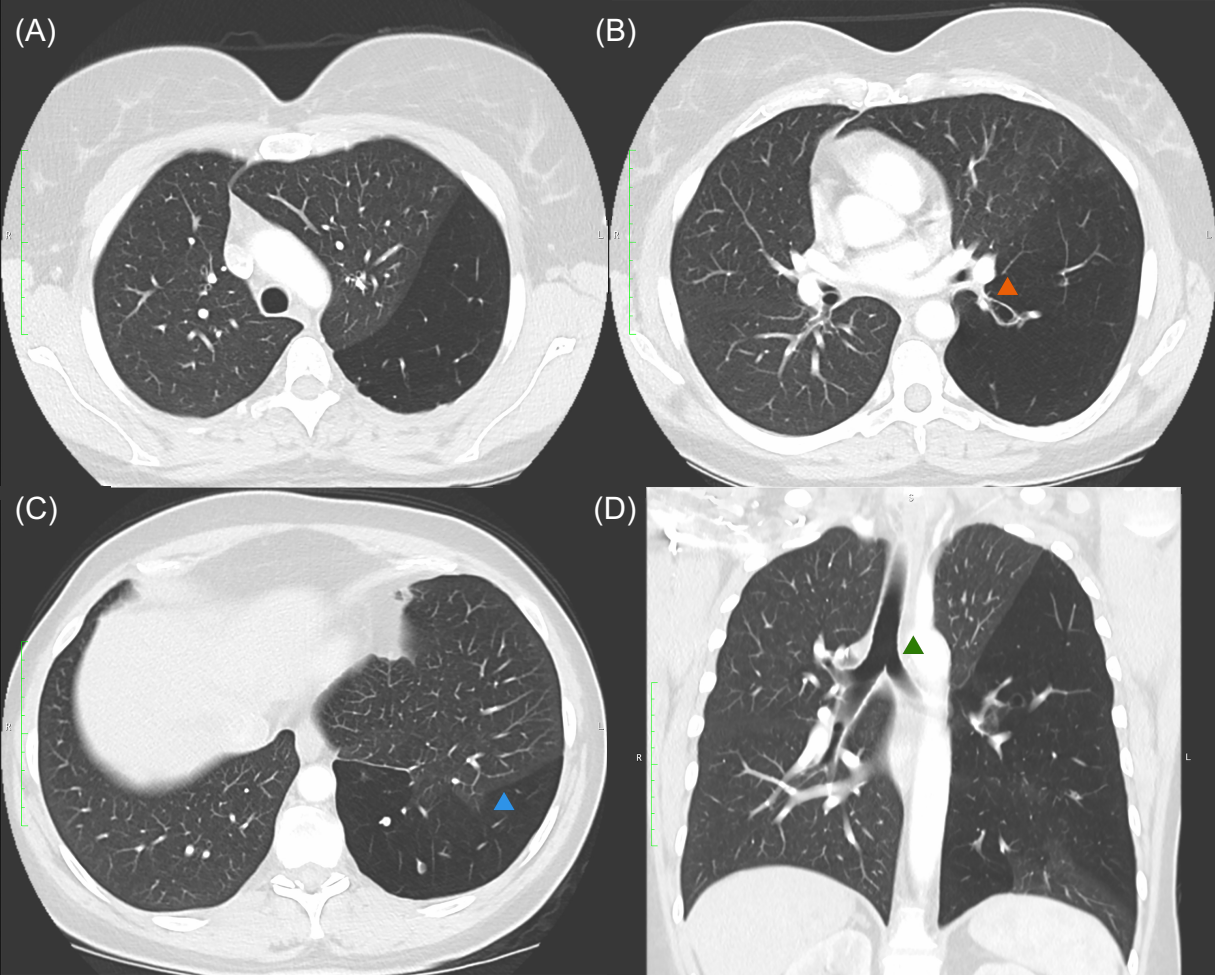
Sagittal and coronal computed tomography images (clockwise from top left; A–D) showing left lower lobe hyperlucency and hypovascularity. (A) demonstrates hyperlucency and hypovascularity of the left lower lobe. (B) demonstrates stenosis at the origin of the left lower lobe bronchus with distal ballooning (orange arrow). (C) demonstrates an incomplete oblique fissure and subsequent parenchymal intrusion into the left lower lobe (blue arrow). In SJMS the volume of the affected lung is normal or, more commonly, reduced. It is seldom if ever increased. In this case collateral air drift through the incomplete fissure is the likely cause of the hyperinflation and subsequent mass effect. (D) demonstrates the degree of mediastinal shift with rightward tracheal deviation (green arrow). Hyperinflation is not typically a feature of SJMS and is often more suggestive of congenital lobar emphysema. If hyperinflation is present, this suggests presence of collateral air flow either from adjacent unaffected lung, or across an incomplete fissure as seen in this case.
